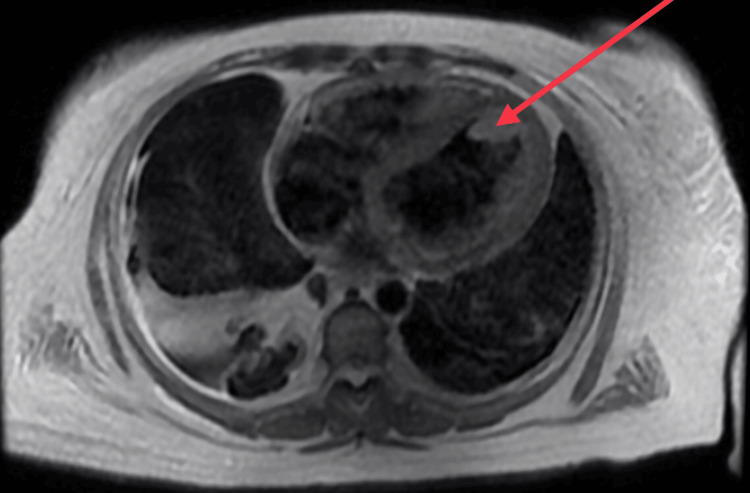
Left ventricular apical thrombus seen on cardiac MRI (arrow)
